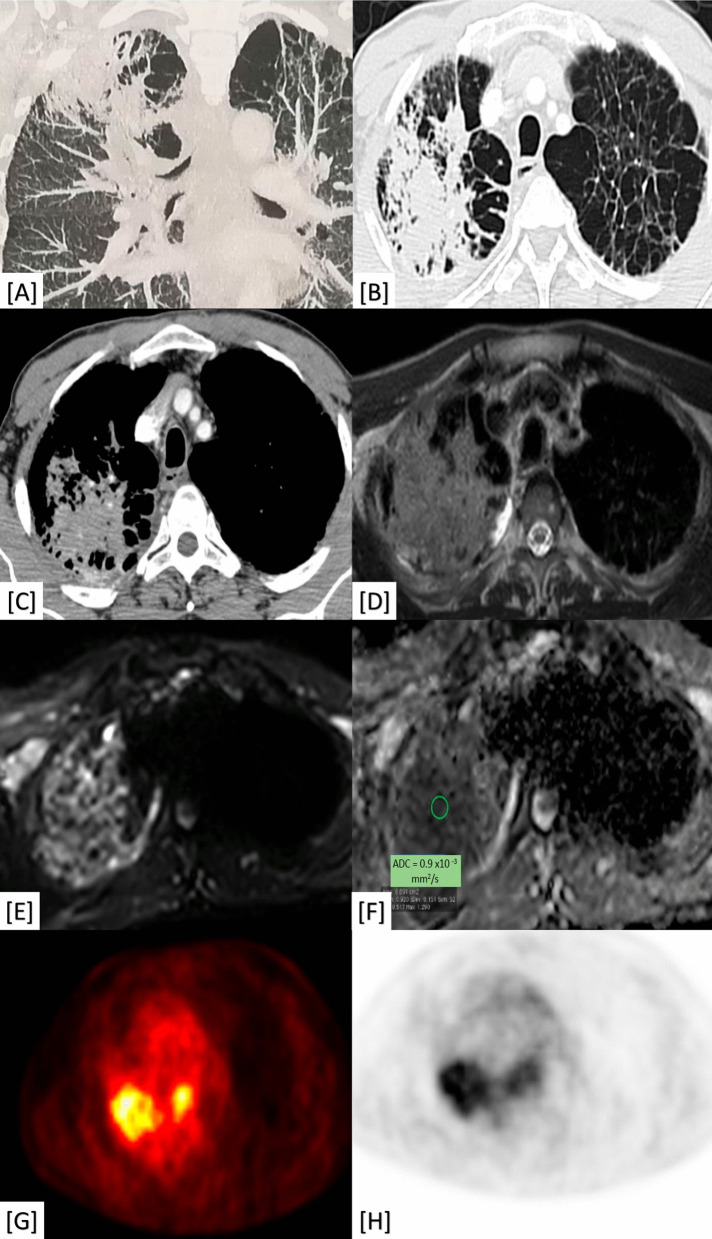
A 58-year-old male heavy smoker patient with a history of old TB complaining of progressive dyspnea. A Initial coronal CT (lung window) showing apical lung scarring. B Follow-up chest CT (lung window) showed progression in size of the scar. C Follow-up chest contrast-enhanced CT (mediastinal window) showing fleshy soft tissue appearance with internal CT angiogram and no air bronchogram. D T2WI MRI revealed a hyper-intense signal of the tumor. E DWI-MRI revealed restriction diffusion. F ADC mapping revealed Low ADC value (0.9 × 10^−3^ mm^2^/s). G, H PET-CT scans showing high FDG uptake. Diagnosis: Proved patient with scar carcinoma sequel to old TB-fibrosis
